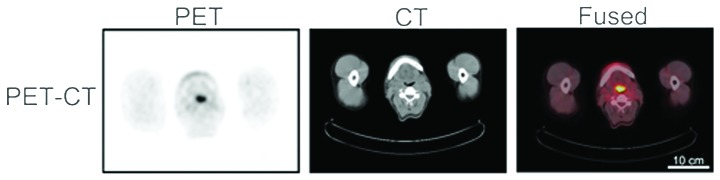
PET, CT and fused images. The tonsil was swollen and the largest standardized uptake value was 8.08 (scale bar, 10 cm). PET, positron emission tomography; CT, computed tomography.
